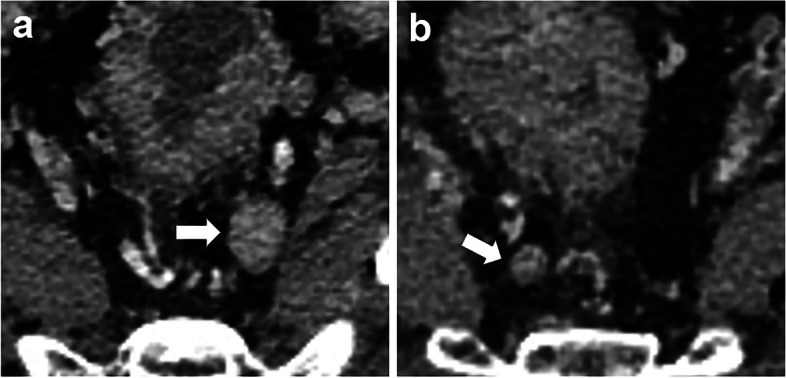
portal venous phase CT images of a 52-year-old male with moderately differentiated pathologic T3N- rectal adenocarcinoma. a The size-prioritized lymph node (indicated by a white arrow) exhibits a short-axis diameter of 12 mm, featuring smooth margins, homogeneous enhancement, and an elliptical shape. It has been assigned a Node-RADS score of 2. b The morphology-prioritized lymph node (indicated by a white arrow) demonstrates a short-axis diameter of 6 mm, displaying clear borders, heterogeneous enhancement, and an elliptical shape. It has been assigned a Node-RADS score of 3. The Node-RADSmax score for this patient is 3
